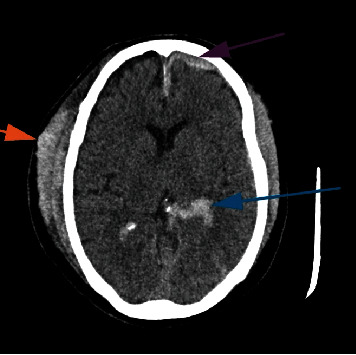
Axial CT scan image showing traumatic hemorrhage (blue arrow, intraventricular; purple arrow, subdural hematoma; orange arrow, scalp hematoma).
